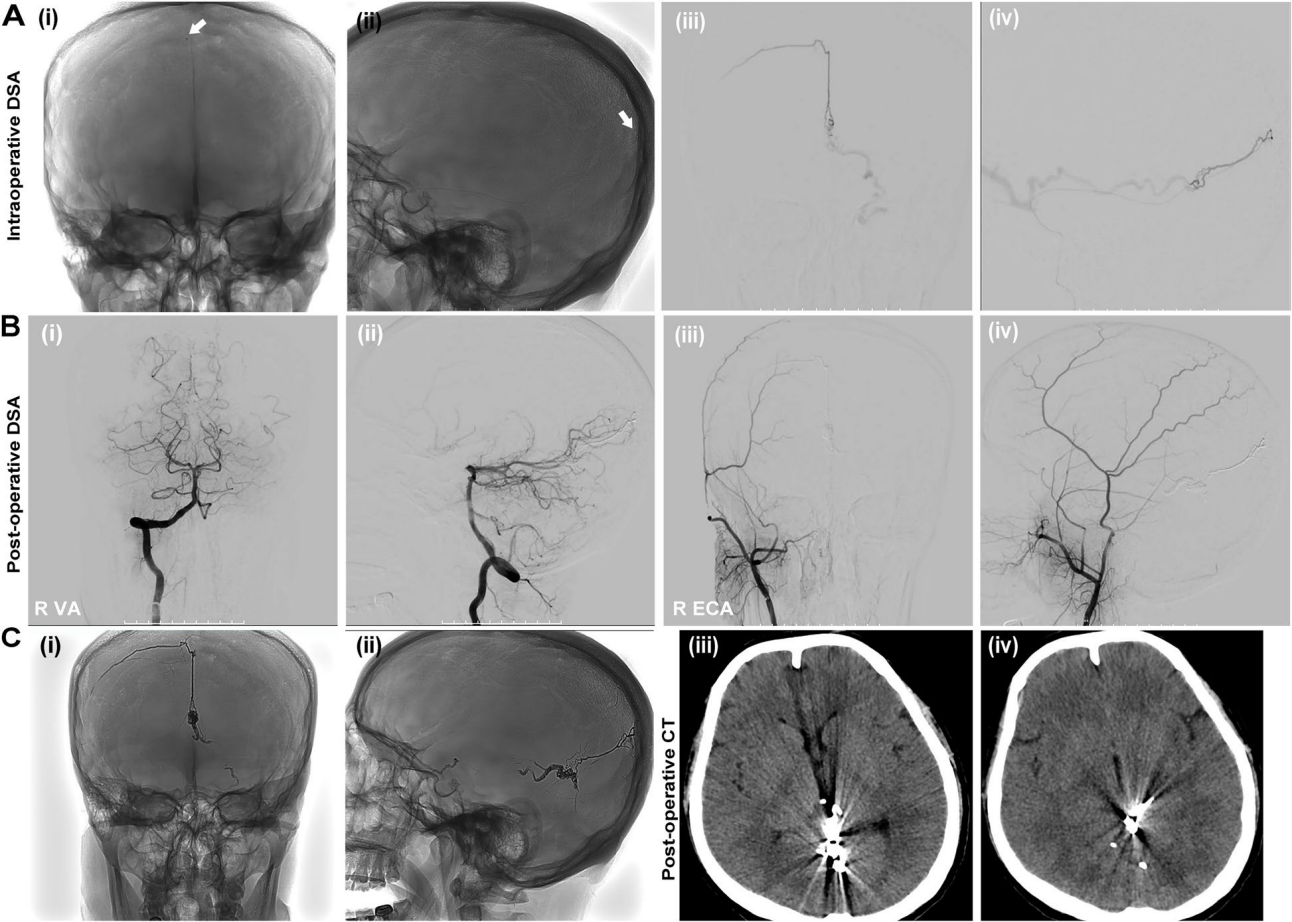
A Intraoperative angiographic imaging showed the position of the microcatheter tip before Onyx injection. (i–ii, white arrow). Angiography was performed via microcatheter (iii, iv). B Post-embolization angiography demonstrated that the DAVF was occluded completely without nontargeted vessel embolization. C Penetration and solidification of Onyx after embolization (i, ii). A postoperative CT scan showed no new cerebral infarction (iii, iv)
